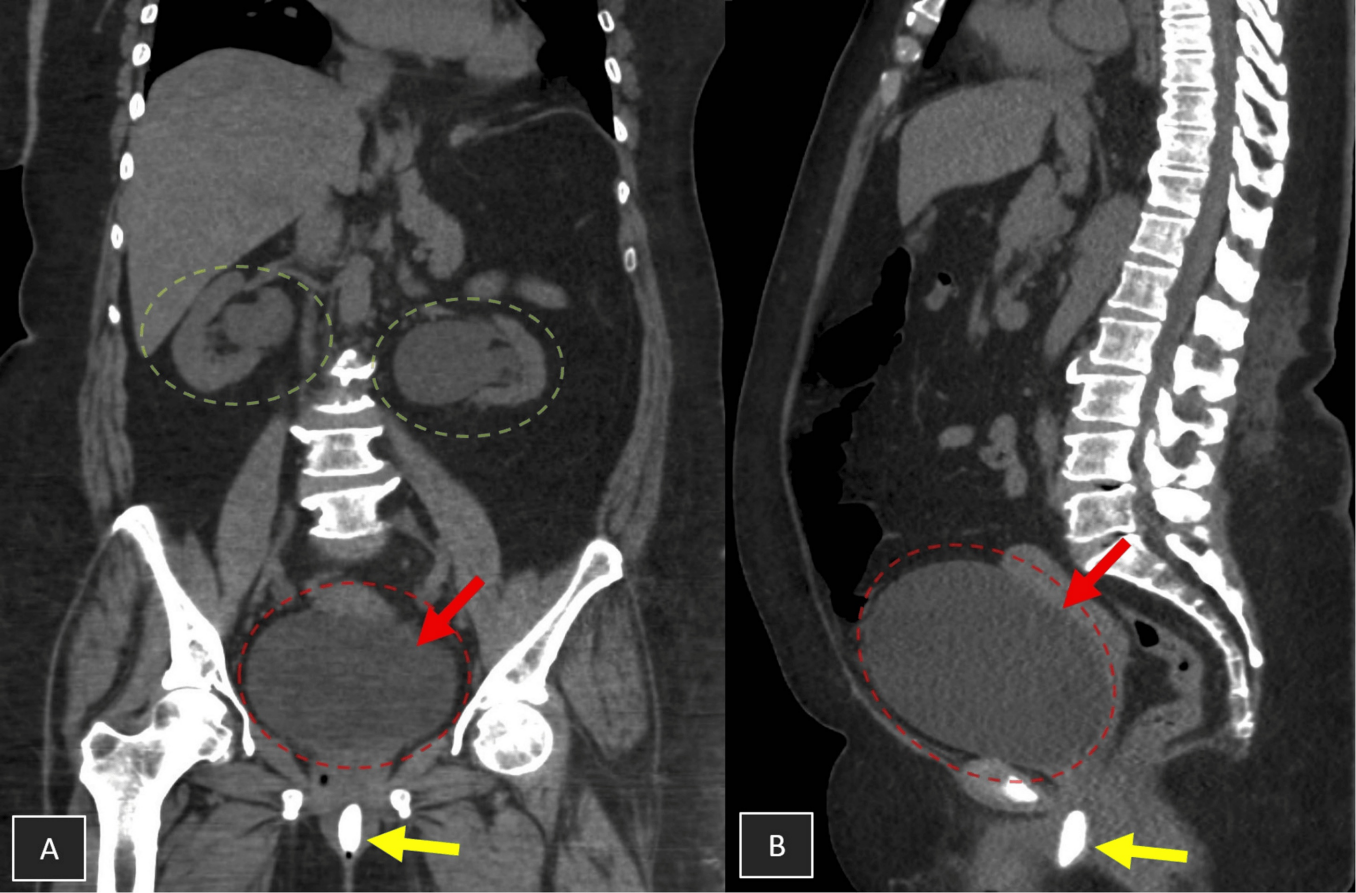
CT KUB showing bilateral hydronephrosis and a distended bladder due to an obstructive urethral stone. A: Coronal view – showing bilateral hydronephrosis (green circles), a distended bladder (red arrow and circle), and a urethral stone (yellow arrow). B: Sagittal view – showing a distended bladder (red arrow and circle) and a urethral stone (yellow arrow). CT KUB: computed tomography of the kidneys, ureters, and bladder
